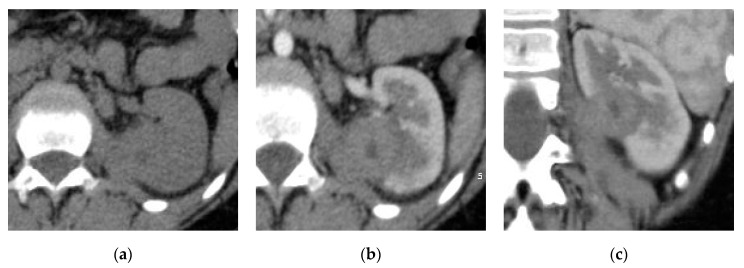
Left hematogenous acute pyelonephritis. (a) Axial non-contrast CT image shows focal contour bulge at the posterior parapelvic region of the left kidney. (b) Axial and (c) coronal reformatted post-contrast CT images show mildly enhancing rounded lesion at the mid-zone of the kidney with adhesion to the left psoas muscle mimicking renal tumor.
